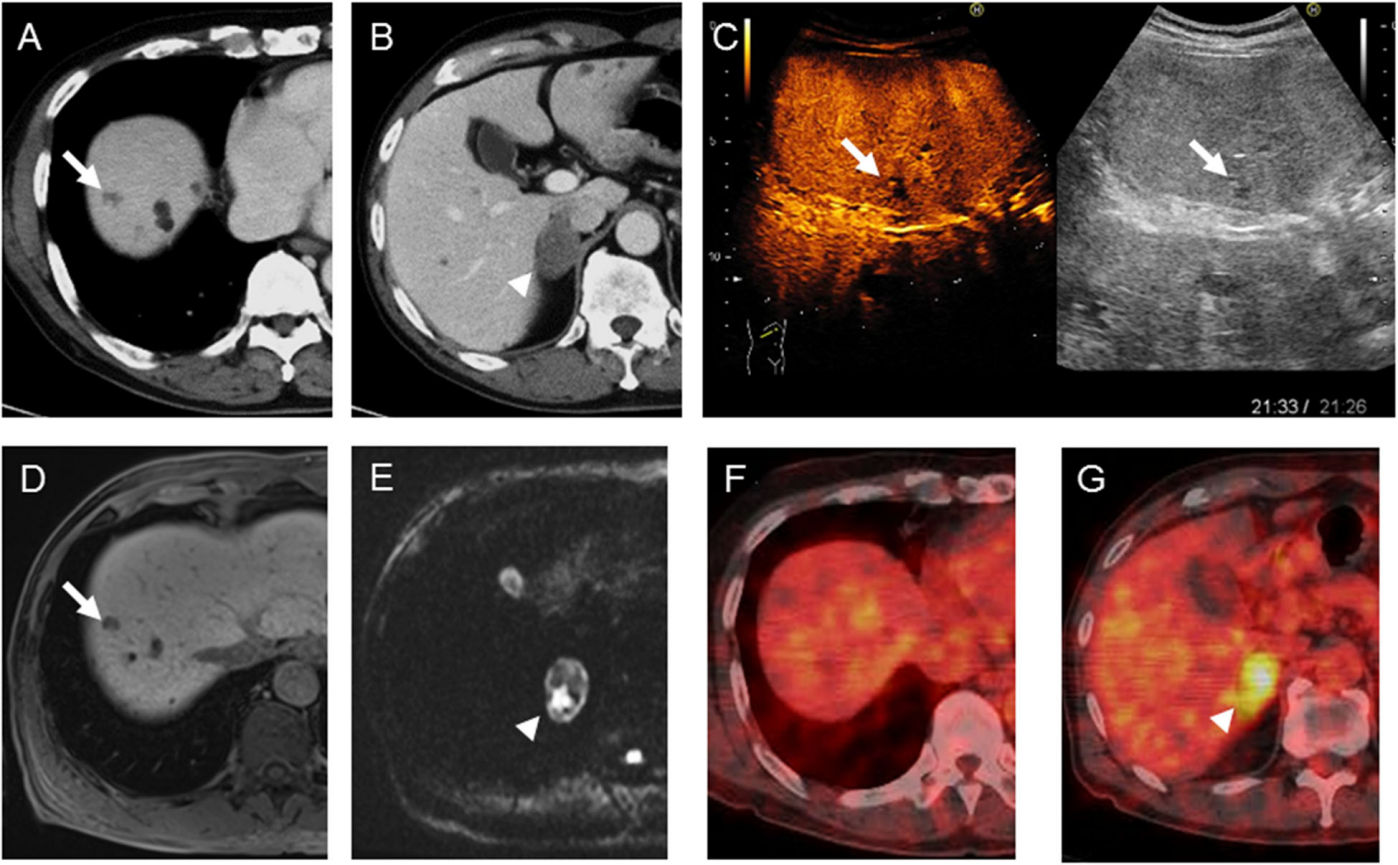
a, b Computed tomography image showing an about 1.5-cm, irregularly shaped mass lesion in segment 8 of the liver (arrow), and about 3.0-cm right adrenal gland tumor having inferior vena cava (IVC) tumor thrombus (arrow head). c Contrast-enhanced ultrasonography image indicating a defect on the Kupffer phase in segment 8 (arrow). d, e Gadolinium-ethoxybenzyl-diethylenetriamine pentaacetic acid magnetic resonance images showing a defect on the hepatobiliary phase at about the liver tumor (arrow) and a high intensity on diffusion-weighted imaging about the right adrenal tumor (arrowhead). f, g
